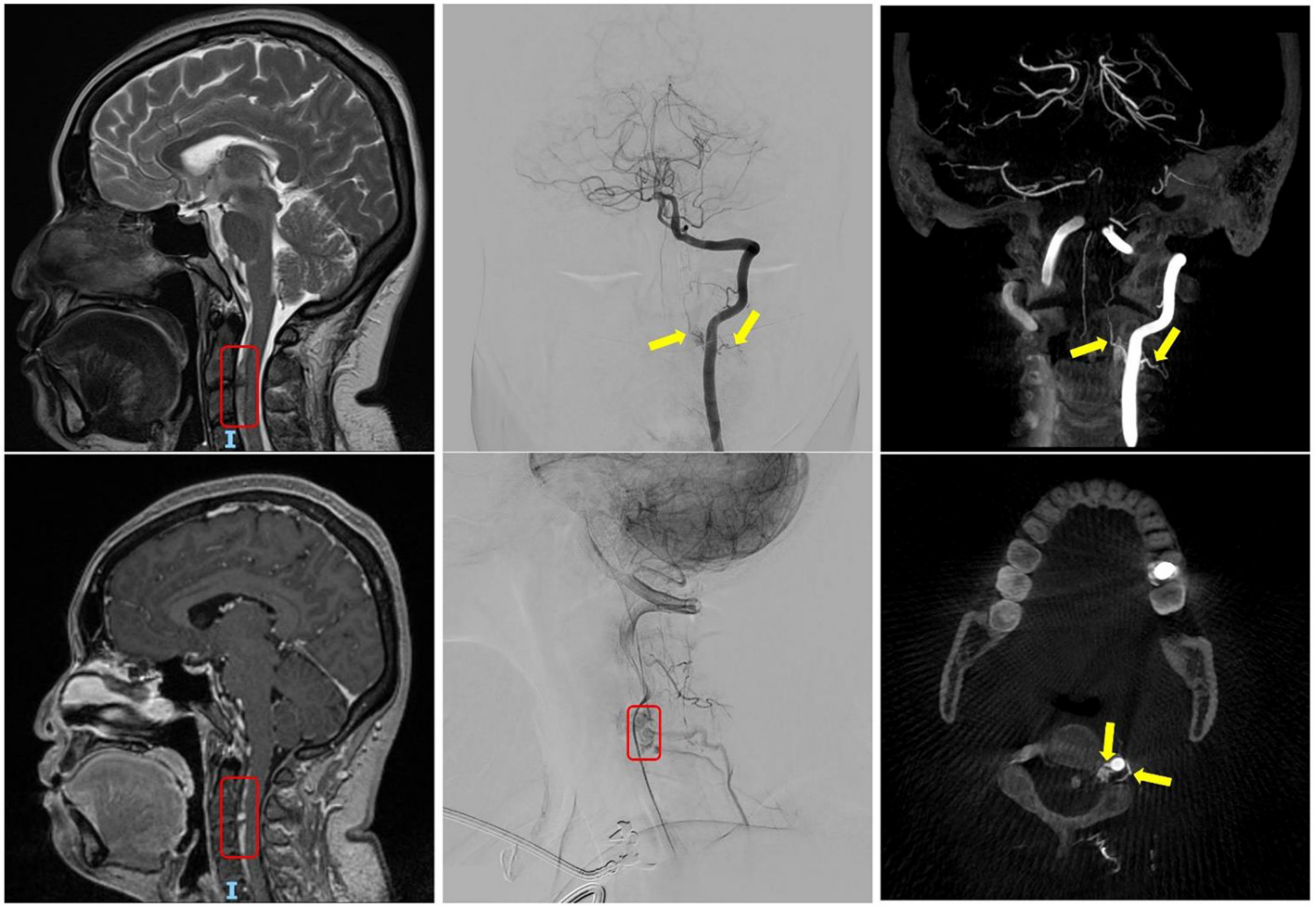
The left panels show the finding of the C2–3 dural arteriovenous fistula (AVF) in routine MRI head, highlighted within the red box. The middle and right panels show the digital subtraction angiography (DSA) cervical spine and the angio-CT of L-VA. The yellow arrows indicate the two supplying arteries; and the red boxes indicate tortuous venous drainage of the AVF.
